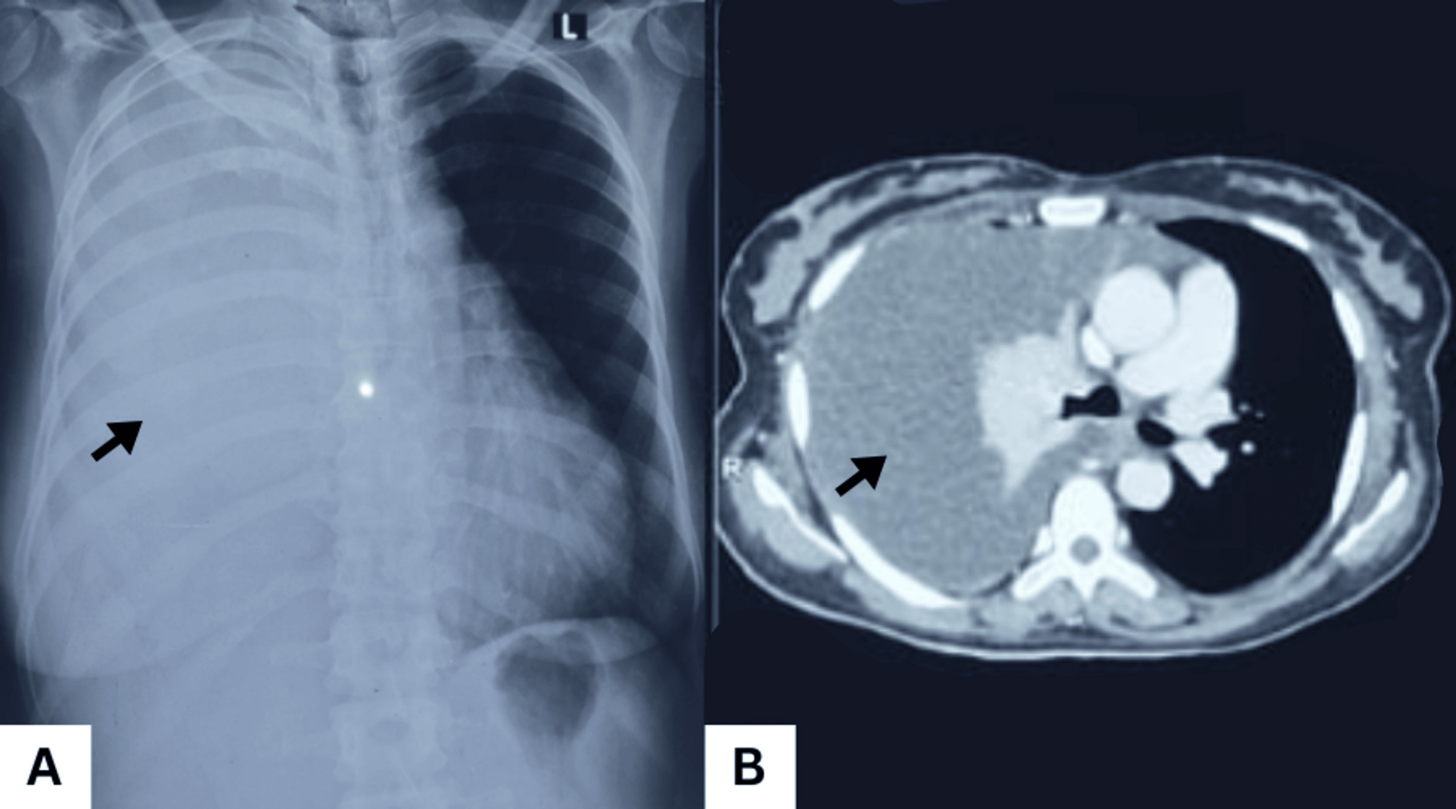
Chest X-ray (A) and CT chest (B) Showing white out right hemithorax with a shift of the mediastinum to the left side, suggestive of massive pleural effusion
